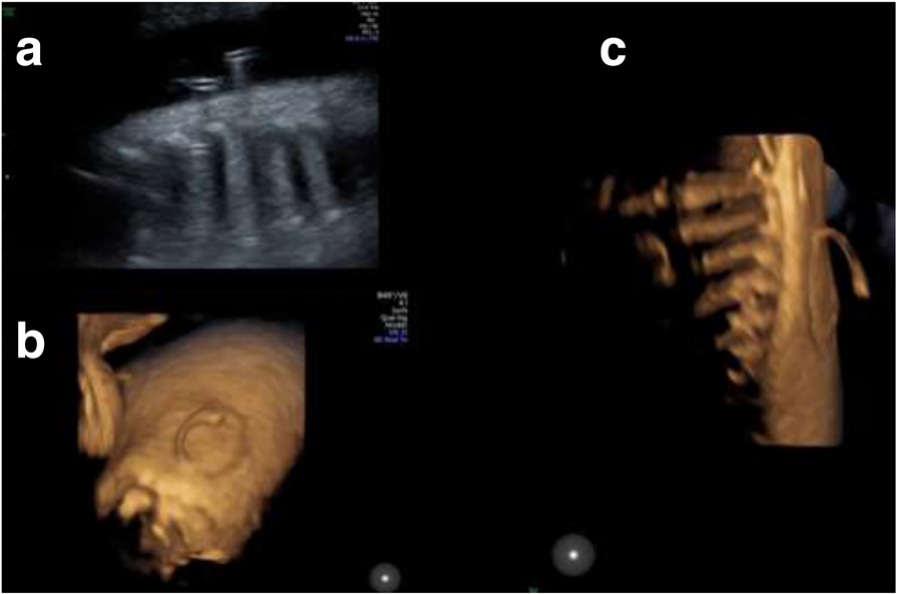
Case 2. 2-D (a) and 4-D (b, c) ultrasound images of the catheter pigtail correctly inserted inside and outside of the fetal hemithorax
